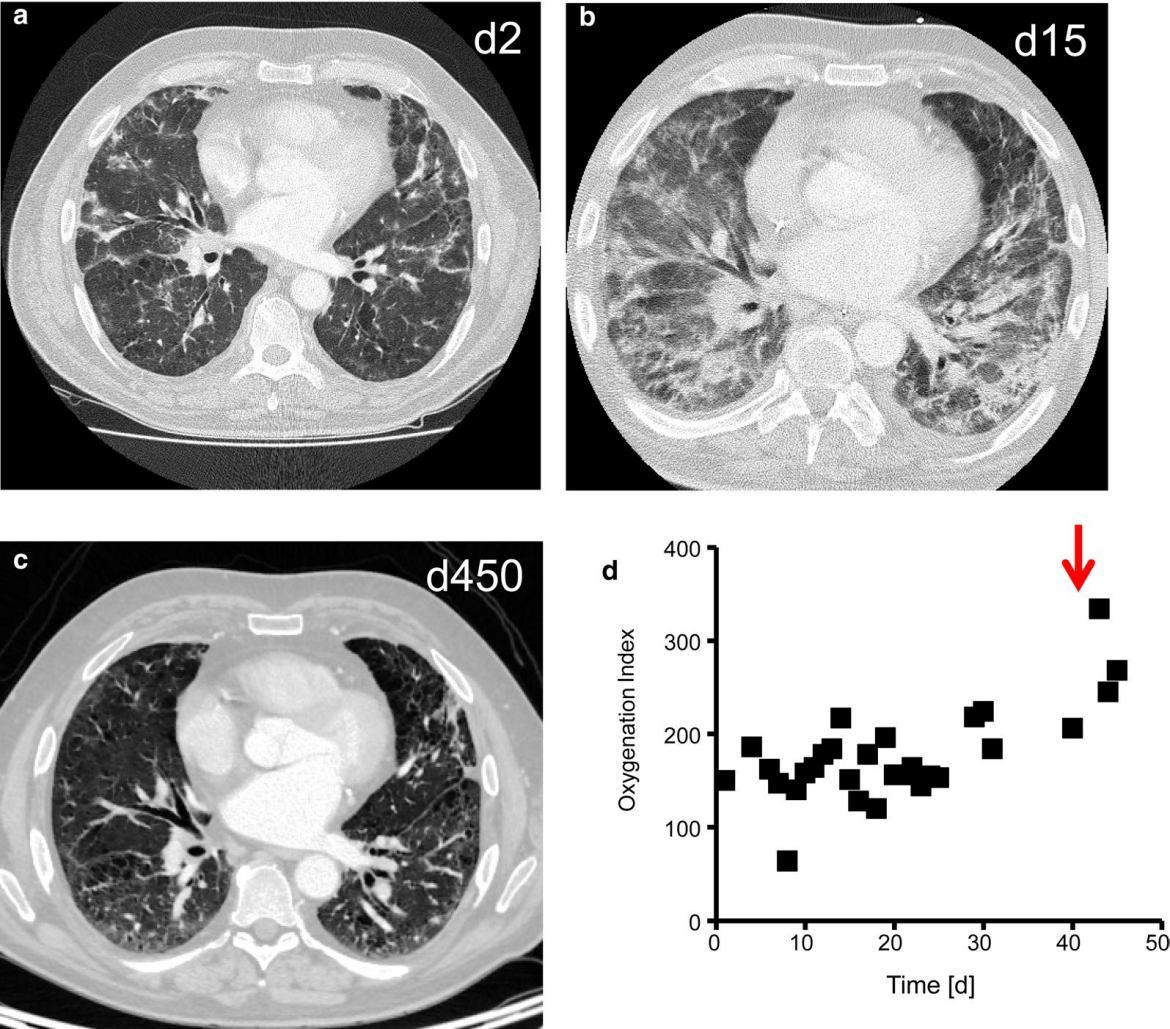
Patient 3. Chest computed tomography showing interstitial pneumonia on day 2 (a), day 15 (b). Immunosuppression with cyclophosphamide (red arrow) was initiated on day 43, causing impressive clinical improvement. A control chest computed tomography was performed after 15 months (c). There was a close temporal association of the initiation of cyclophosphamide (red arrow) on day 43 with recovery of oxygenation index (d)
